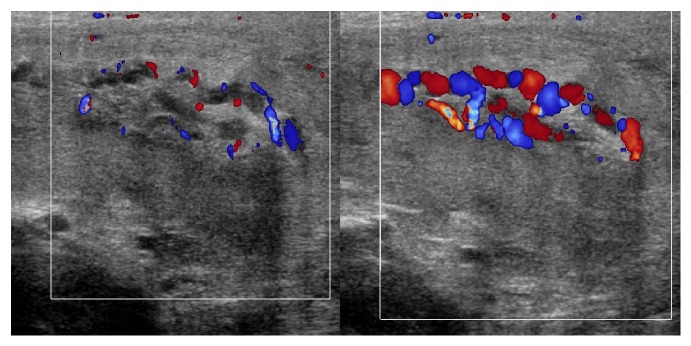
Doppler ultrasonography: dilatation of the pampiniform plexus of veins, image compatible with the varicocele.
